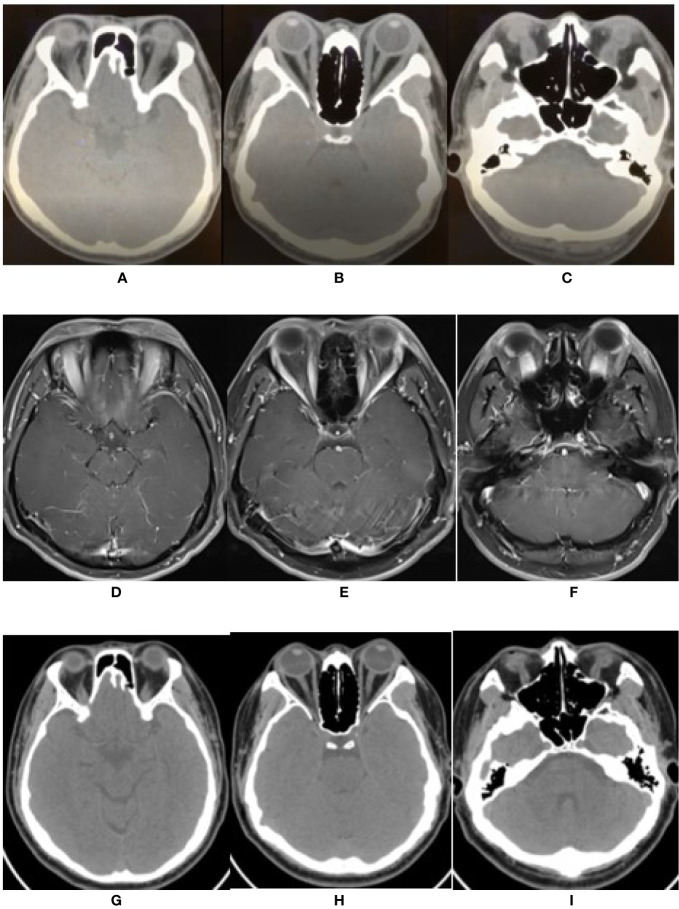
The changes of imaging of extra-ocular muscles. (A–C) Orbital CT scan before therapy showed the bilateral ophthalmic muscles including superior rectus, medial and lateral rectus, inferior rectus were obviously thickened. (D–F) Enhanced MRI revealed thickened and enhanced multiple extra-ocular muscles including tendinous insertion. (G–I) After 3 months of treatment, orbital CT showed bilateral extra-ocular muscles were not thickened.
